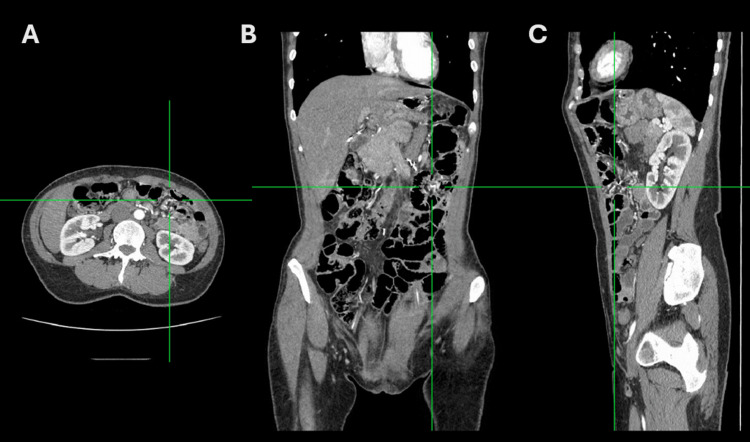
Abdominal CT scan Contrast-enhanced abdominal CT images. Axial (A) and coronal (B) views demonstrate clustering of small bowel loops predominantly in the left abdominal flank. Although no definitive radiological signs of internal herniation, such as the whirlpool sign, are identified, these findings are considered non-specific but clinically suspicious in the context of the patient’s symptoms, thereby contributing to the decision for surgical exploration. Axial (A), coronal (B), and sagittal (C) views additionally show a mildly dilated hepatobiliary limb containing fluid, most consistent with bile.
